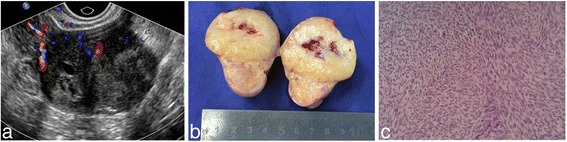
A 53-year-old pre-menopausal woman having a pelvic mass was detected by physical examination. a On ultrasound examination, a 28 mm*22 mm*26 mm well-demarcated hypoechoic mass with small anechoic areas was observed at left ovary, and moderate Doppler signals were seen in the tumor; b the tumor section showed grey-white appearance and yellowish areas with hemorrhage in the center of the tumor; c pathological findings proved to be cellular fibroma with partial growth activity
